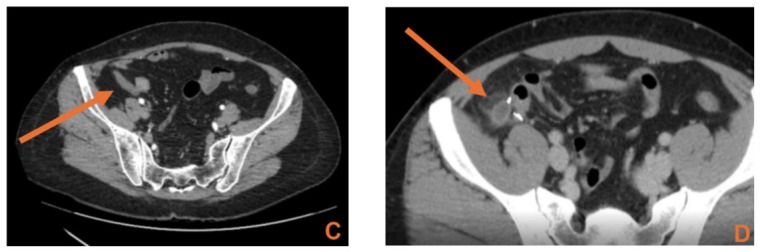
MRI and CT findings in rare appendicular pathologies. (A) Pelvic MRI showing a dilated appendiceal base suggestive of a mucocele (arrow). (B) CT scan of a mucinous cystadenoma demonstrating appendiceal dilatation with mural calcifications (arrow; R marks the right side of the patient). (C) CT scan showing nonspecific findings in appendicular diverticulum (arrow). (D) CT scan demonstrating stump appendicitis, with previously placed clips at the appendiceal base and appendicular artery (arrow).
